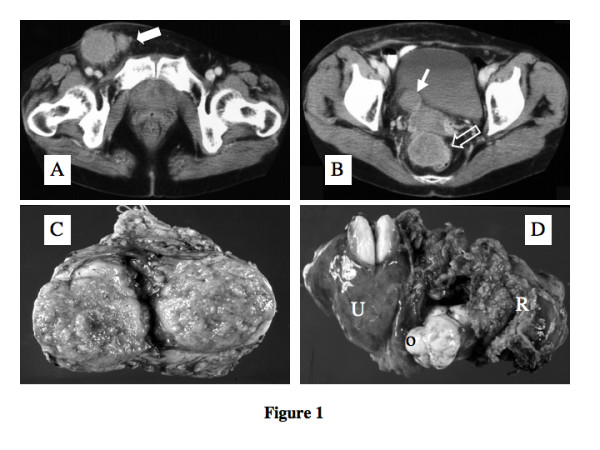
A. CT demonstrated a large lump of inguinal tumor (arrow) on the right femoral vessels. B. CT showed tumors with 3 cm in diameter locates between urinary bladder and uterus (solid arrow) and a bigger (5 cm) tumor (hallow allow), showing invasive growth to the adjacent uterus and rectum. C. Right inguinal tumor is circumscribed fairly well and measured 42 × 25 × 12 mm in size. The cut surface is swell up and reddish-white, and had hemorrhagic and necrotic foci. D. A lump of intra-pelvic tumors removed by total hysterectomy (U) with salpingo-oophorectomy and a partial resection of the rectum wall (R) at the rectosigmoid junction.
